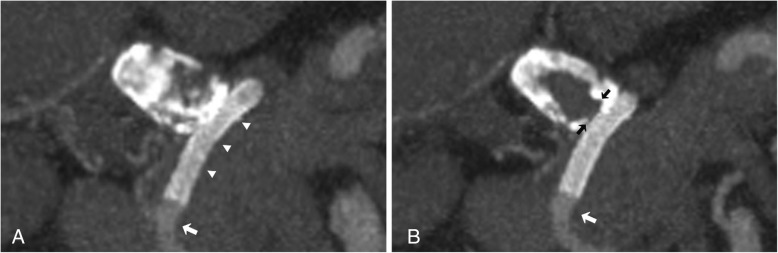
8-week CTA follow up. a) and b) Coronal maximum intensity projection (MIP) CTA demonstrating patency of the Surpass Streamline flow diverting stent (arrowheads) and preservation of flow into the distal gastroduodenal artery (arrow). Note complete thrombosis of the aneurysm with no residual neck (black arrows)
